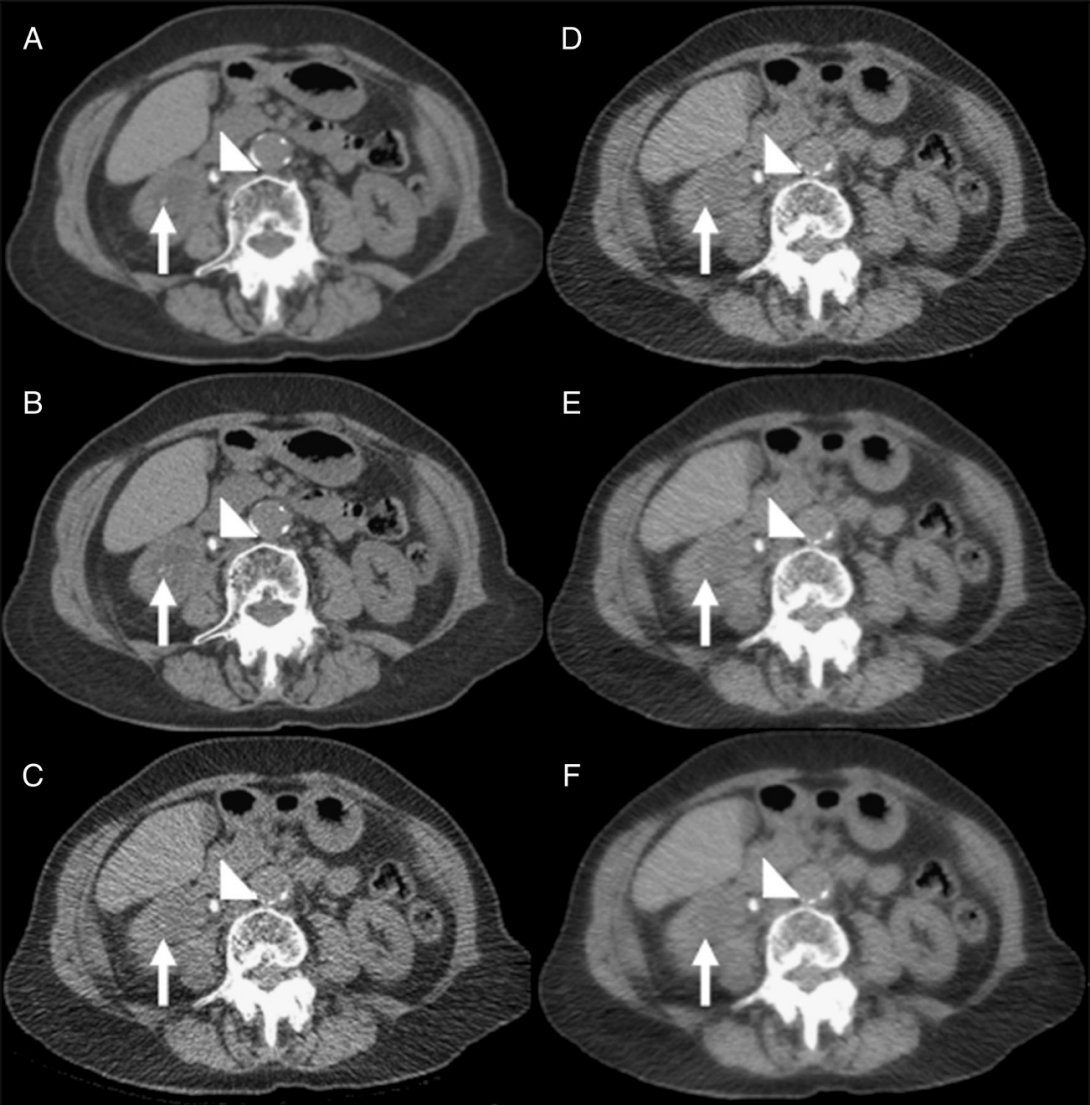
A 48-year-old female patient (BMI = 25.6) with acute right ureteric colic. a and b Axial conventional dose (5.1 mSv) non-contrast CT image reconstructed with 40 % ASiR (a) and 100 % FBP (b) showing an 8-mm right ureteric calculus (*arrowhead*) and an additional 2-mm renal calculus in the right lower pole (*arrow*) that was not prospectively detected on the low-dose images. c-f Axial low-dose (0.56 mSv) non-contrast CT image reconstructed with 100 % FBP (c), 40 % ASiR (d), 70 % ASiR (e) and 90 % ASiR (f) showing the obstructing 8-mm calculus in the proximal right ureter (*arrowhead*). The location of the smaller 2.1-mm calculus is also demonstrated (*arrow*). Note the ‘over smoothening’ of the soft tissues on the 90 % ASiR image compared to the other low-dose reconstructions
